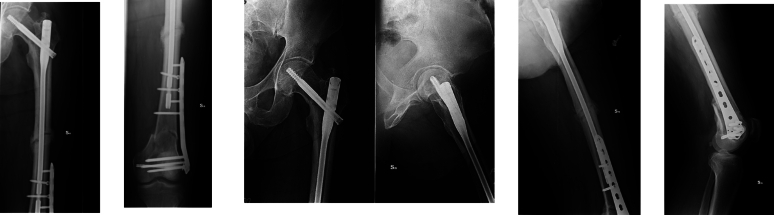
Control X-ray four months after surgery.
